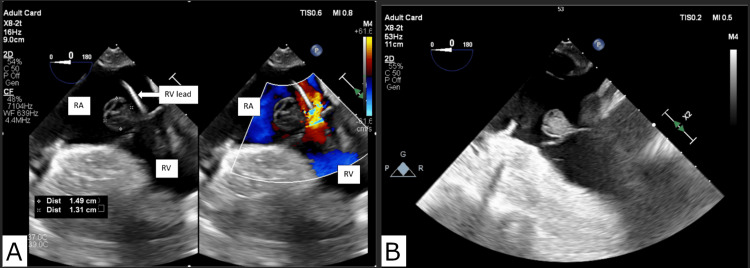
Intraoperative transesophageal echocardiogram (TEE). A) Vegetation prior to pacemaker lead extraction and B) after pacemaker lead extraction. RA: right atrium; RV: right ventricle
